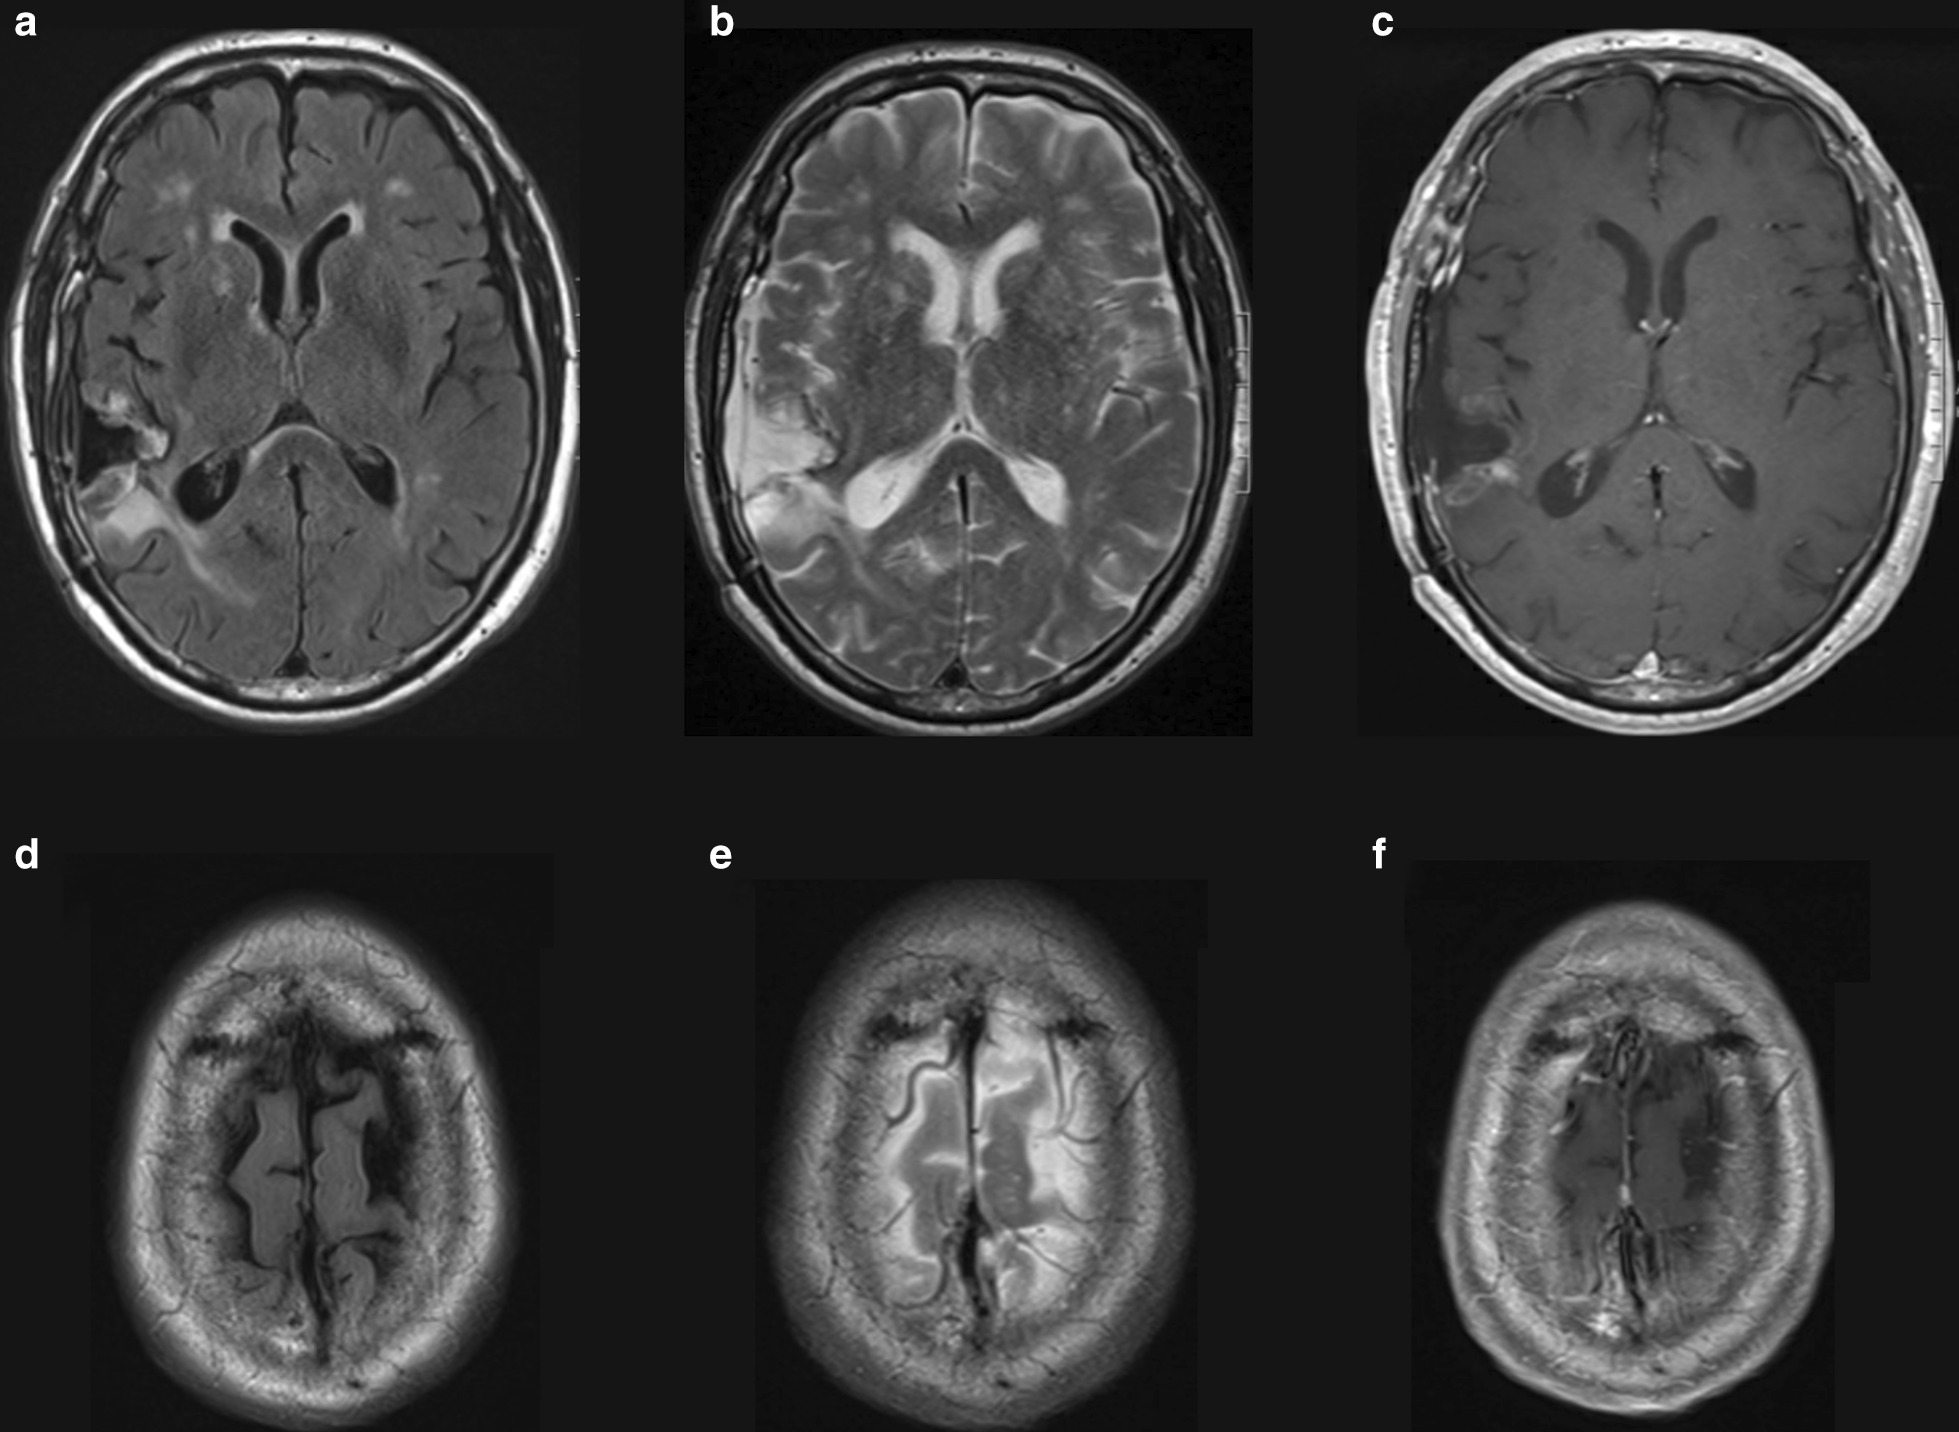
Magnetic resonance imaging of the brain 4.5 months postoperatively. Fluid-attenuated inversion recovery (FLAIR) (a), T2 (b), and T1 (c) show mild irregular rim of enhancement around resection. Resolution of previously seen mass effect and midline shift. Decreased surrounding T2/FLAIR hyperintense signal change. FLAIR (d), T2 (e), and T1 (f) images showing interval resolution of previously noted 5 mm enhancing lesion in the right superior frontal gyrus
